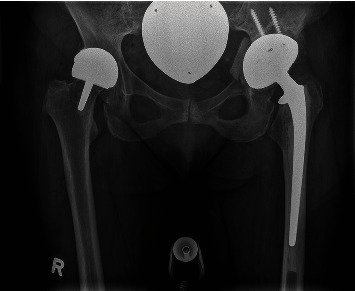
Follow-up X-ray 6 months after surgery. The anteroposterior view of the pelvis shows the correct placement of the implants and a boney healing of the Os ilium.
